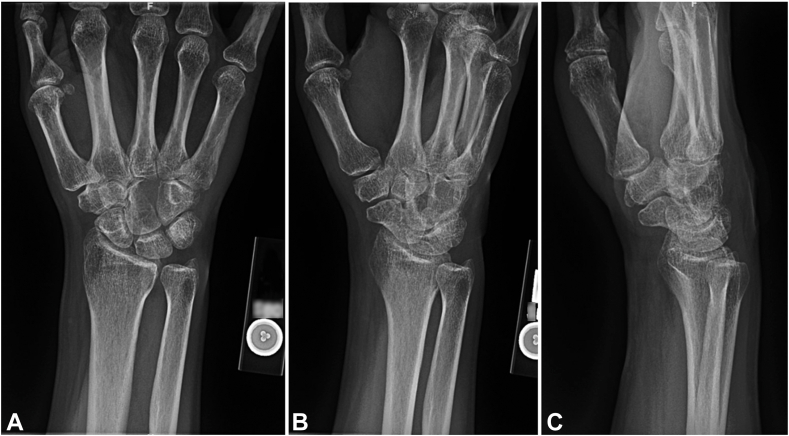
A–C Plain radiographs of the wrist demonstrating destructive osteolytic lesion of the capitate and hamate.
